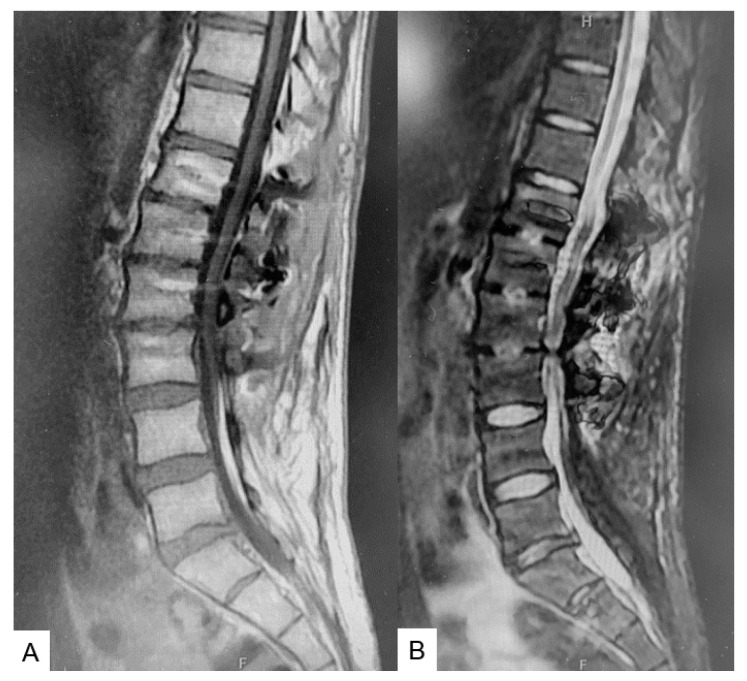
Follow-up images. (A) Mid sagittal T1-weighted MR imaging. (B) Mid sagittal T2-weighted MR imaging.
